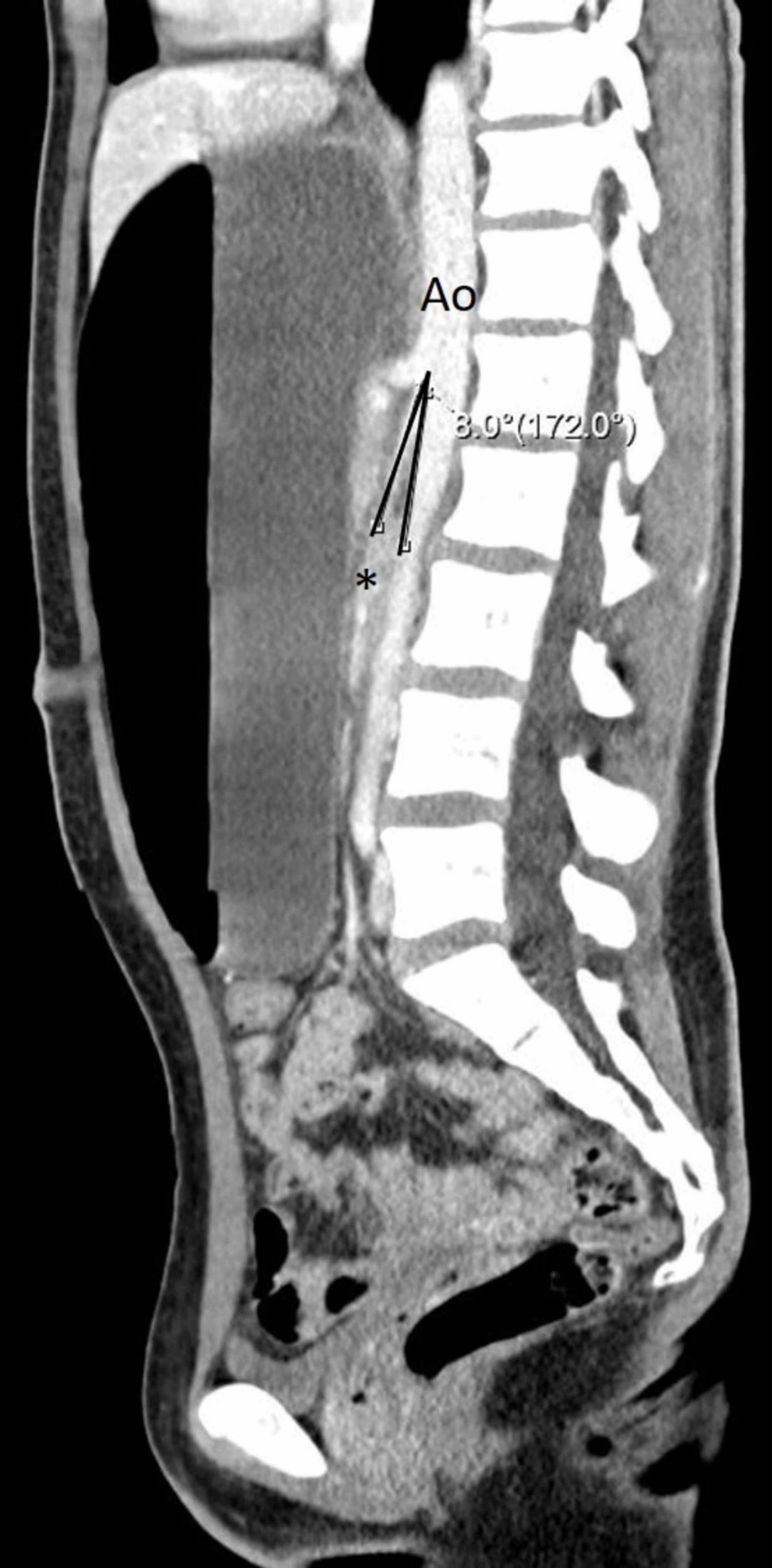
IV contrast-enhanced sagittal computed tomography (CT) of the abdomen and pelvis showing a reduced aortomesenteric angle of 8.0 degrees consistent with superior mesenteric artery syndrome. Ao: aorta, asterisk: superior mesenteric artery.
